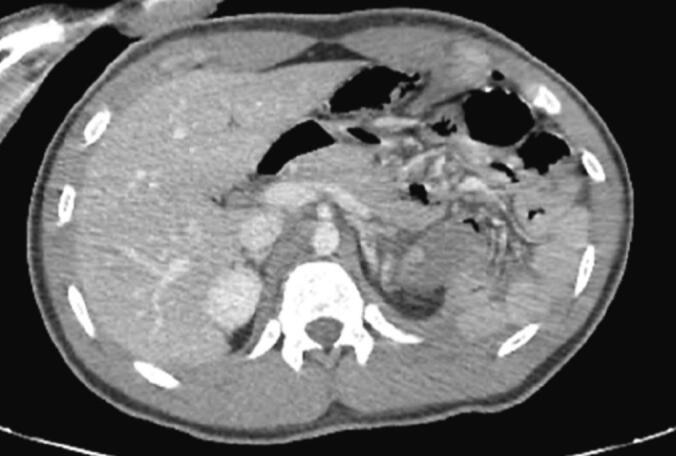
Axial CT section showing a heterogeneous spleen fracture.
